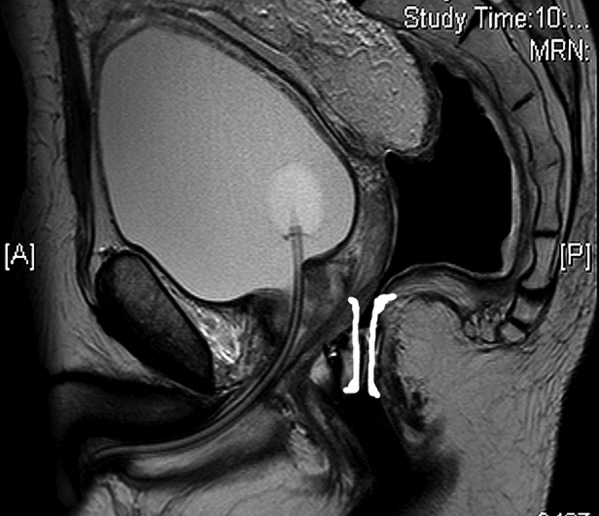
Sagittal magnetic resonance imaging of pelvis five days after the insertion of the Heald stent (highlighted) showing urinary catheter in the bladder and complete drainage of the presacral collection, now seen as air
